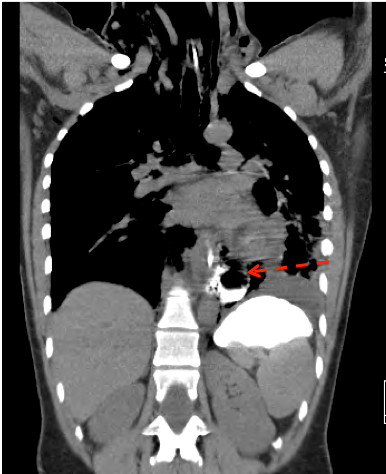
Computer tomography (CT) with an oral contrast swallow, showing distal contrast leakage and gas bubbles in the mediastinum only few hours after pneumatic dilatation for achalasia.
